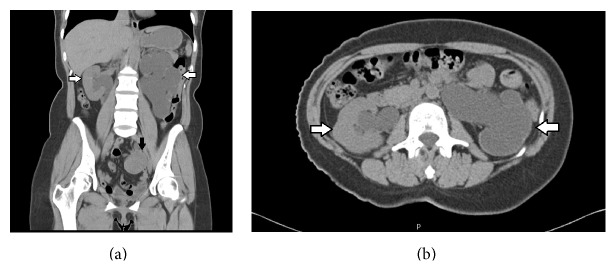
Pelvic CT (computed tomography) showed 4.5 cm sized left adnexal mass (black arrow in (a)) abutting with and obstructing the left distal ureter, resulting in severe left hydroureteronephrosis with complete loss of the left renal parenchyma; it also showed right mild hydroureteronephrosis without definite obstructive lesion (white arrows in (a) and (b)).
